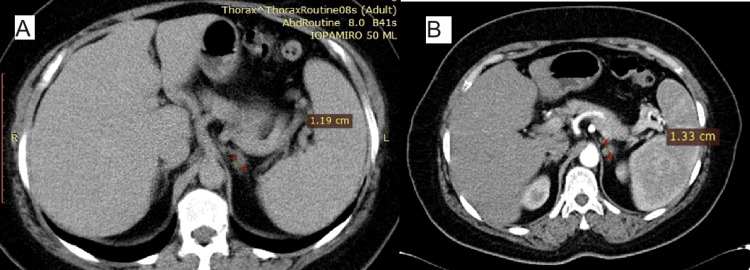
CT scans of the left adrenal mass Arrows showing the adrenal tumour dimensions in consecutive evaluations, year 2010 (Panel A) and year 2011 (Panel B)
